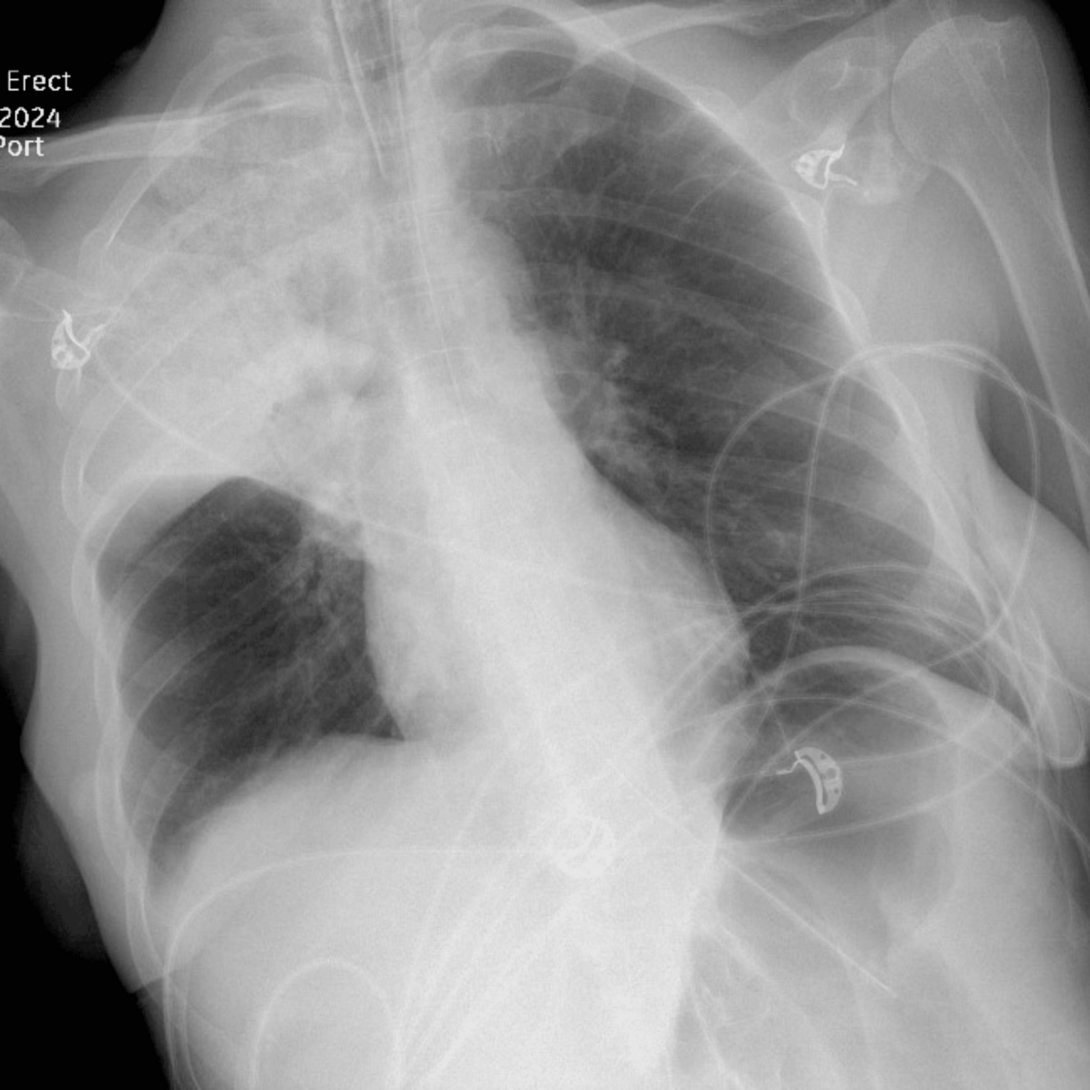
Radiograph of the chest obtained post-bronchoscopy showing some resolution of apical consolidation.
